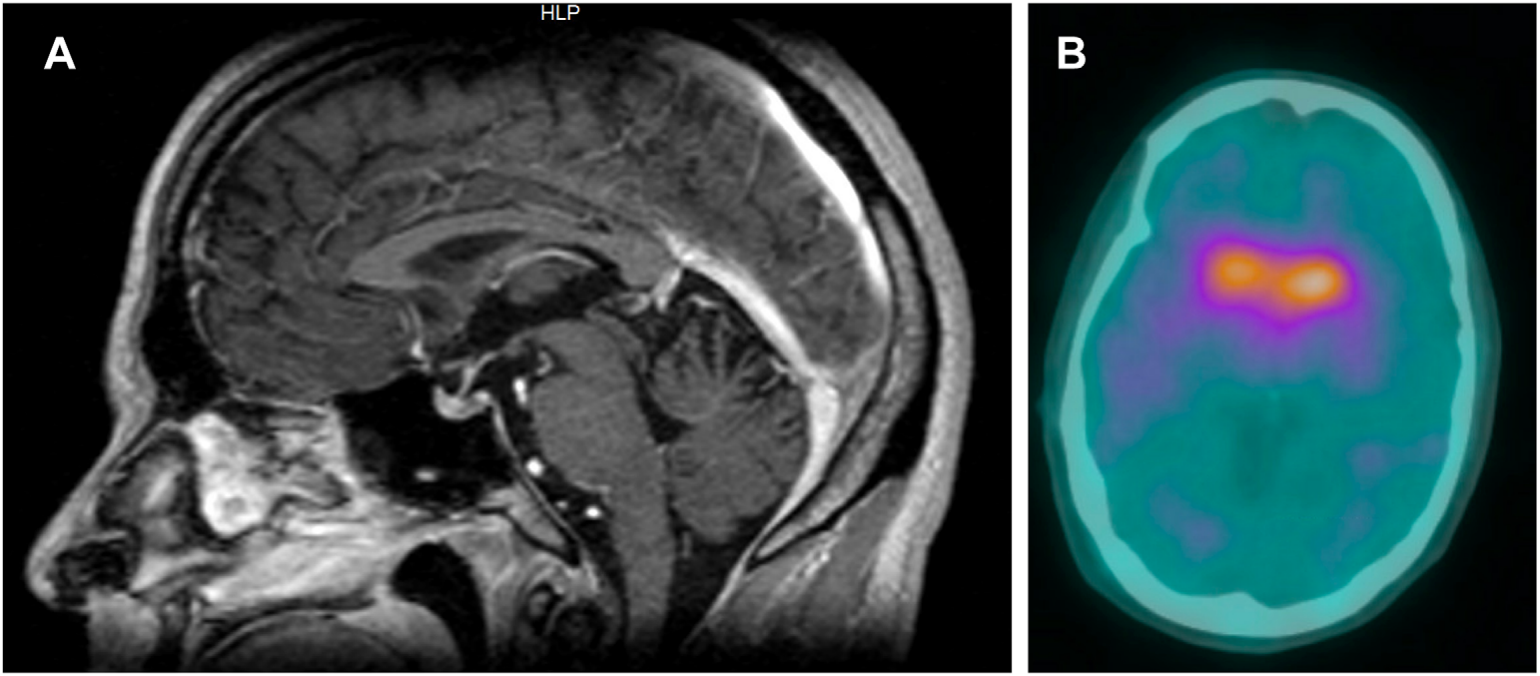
MRI brain and Ioflupane I‐123 SPECT imaging. (A) Brain MRI sagittal T1 demonstrated midline cerebellar atrophy. (B) Ioflupane I‐123 SPECT imaging shows decreased radiotracer uptake within the posterior putamen bilaterally.
